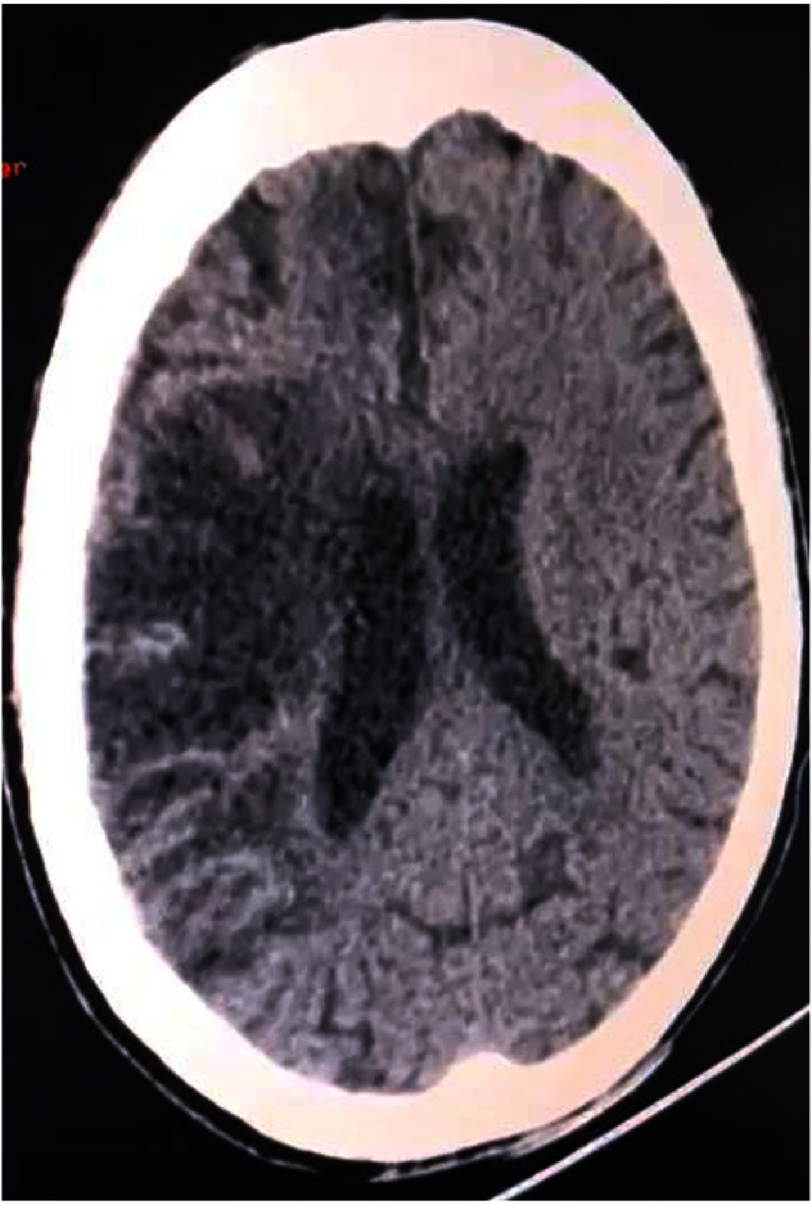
Brain CT showing a large hypodense cortico-subcortical lesion in the right MCA territory, consistent with an acute ischemic stroke, without evidence of hemorrhage.
